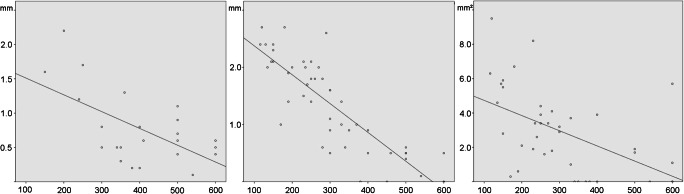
Correlation between intrastenotic diameter/cross-sectional area and peak systolic velocity of internal carotid artery stenosis. Correlation between the narrowest intrastenotic diameter (assessed by digital subtraction angiography (a) and CT angiography (b)), respectively, the narrowest intrastenotic cross-sectional area (assessed by CT angiography (c)) and the peak systolic intrastenotic velocity (*x*-axis, in cm/s) measured by colour-coded duplex sonography
